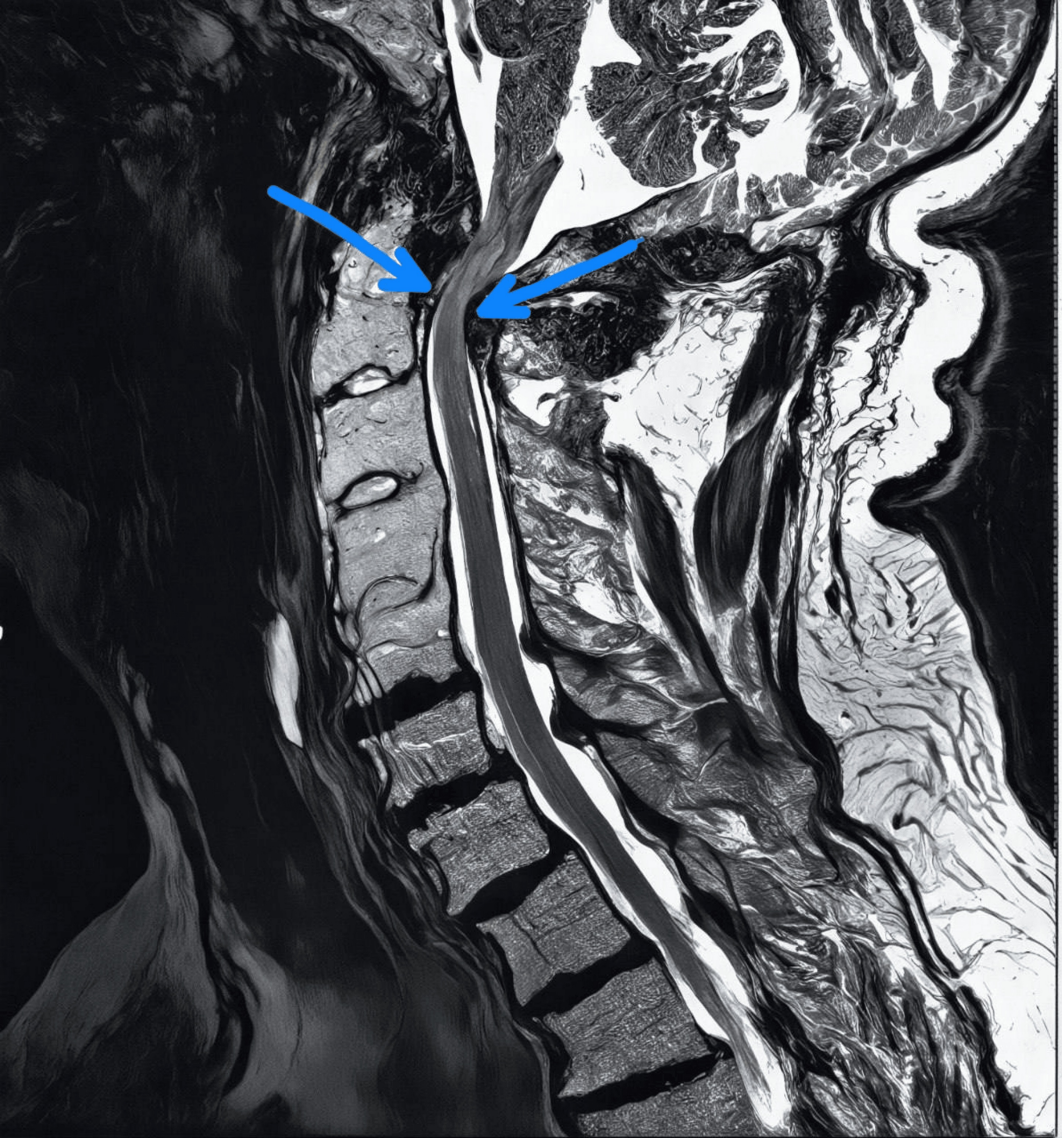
Cervical spine MRI Sagittal T2-weighted MRI of the cervical spine highlighting significant stenosis at the C1-2 junction, marked by arrows indicating both anterior and posterior compression.
